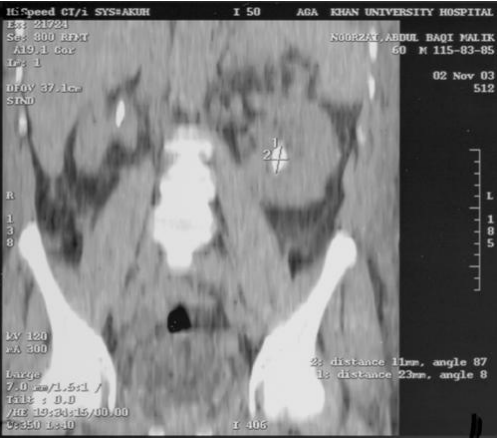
Reformatted unenhanced helical CT image of the same patient showing small-scared right kidney with proximal ureteric calculus and hydroureter. Left kidney shows hydronephrosis and renal calculus.
